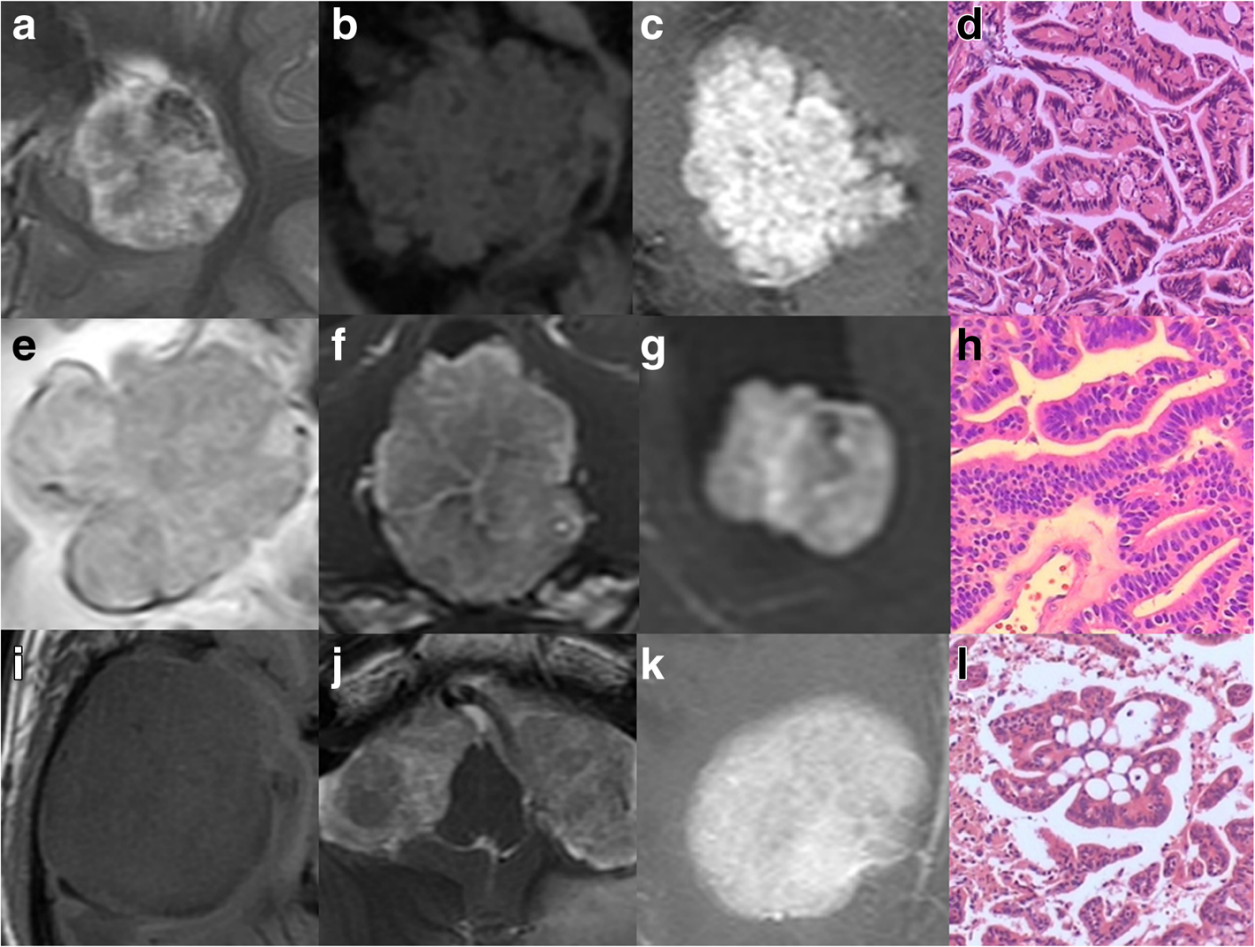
The CPTs with typical papillary/lobulated/irregular internal morphology. The CPPs with papillary internal morphology (a. T2WI, b. T1WI, c. post-contrast). In the pathology image of case b, the tumour cells are arranged in a papillary structure with vascular at the centre (d). The CPC (e. FLAIR) and aCPPs (f, g. post-contrast) with lobulated internal morphology. In the pathology image of case e, the tumour cells are arranged closely, with focal necrosis and haemorrhage (h). The CPCs with irregular internal morphology (i. FLAIR, j. T2WI, k. post-contrast). In the pathology image of case i, the papillary structure is irregular and complex, and the distribution of tumour cells is dense (l)
